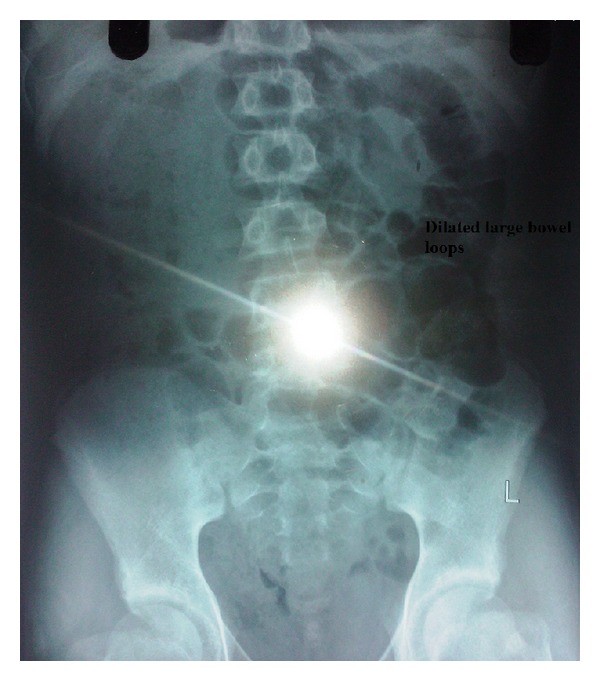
Erect abdominal X-ray showing dilated large bowel loops.
